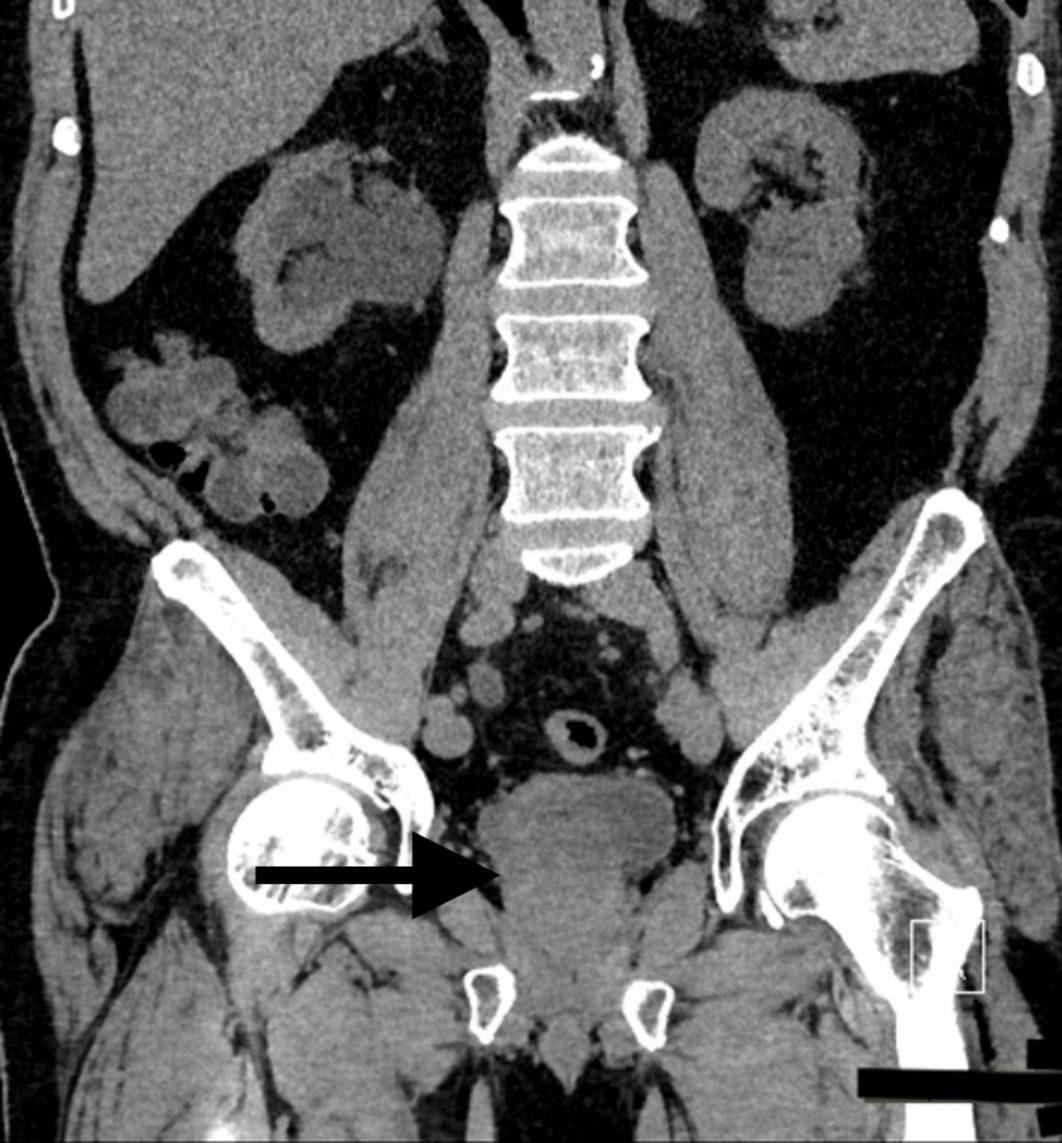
CT abdomen and pelvis coronal view showing bladder mass
